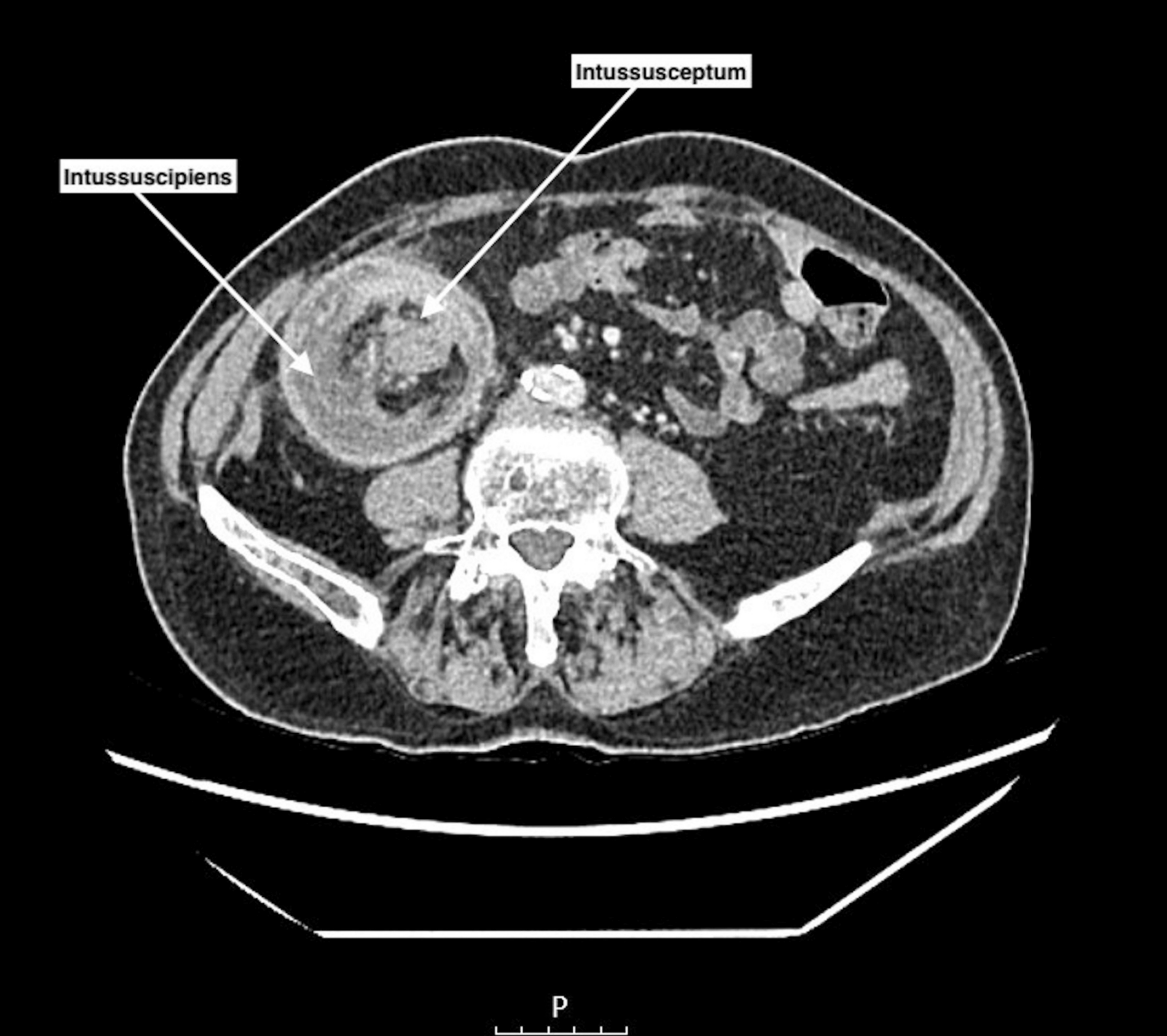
Axial reconstruction of a CT abdomen and pelvis with contrast showing intussusception. Axial CT image shows the 'bowel within bowel' configuration of the proximal part of the intussusception: the intussusceptum centrally is surrounded by mesenteric fat, which is contained within the ring-like intussuscipiens (recipient segment).
